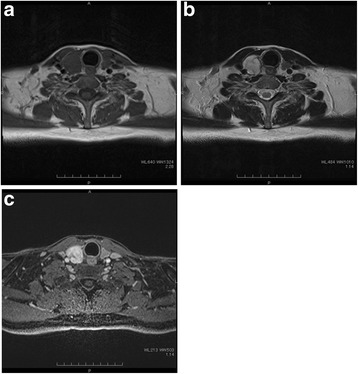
Magnetic resonance T1-weighted image (a), T2-weighted image (b), and enhanced image with fat suppression (c) of metastatic thyroid tumor
